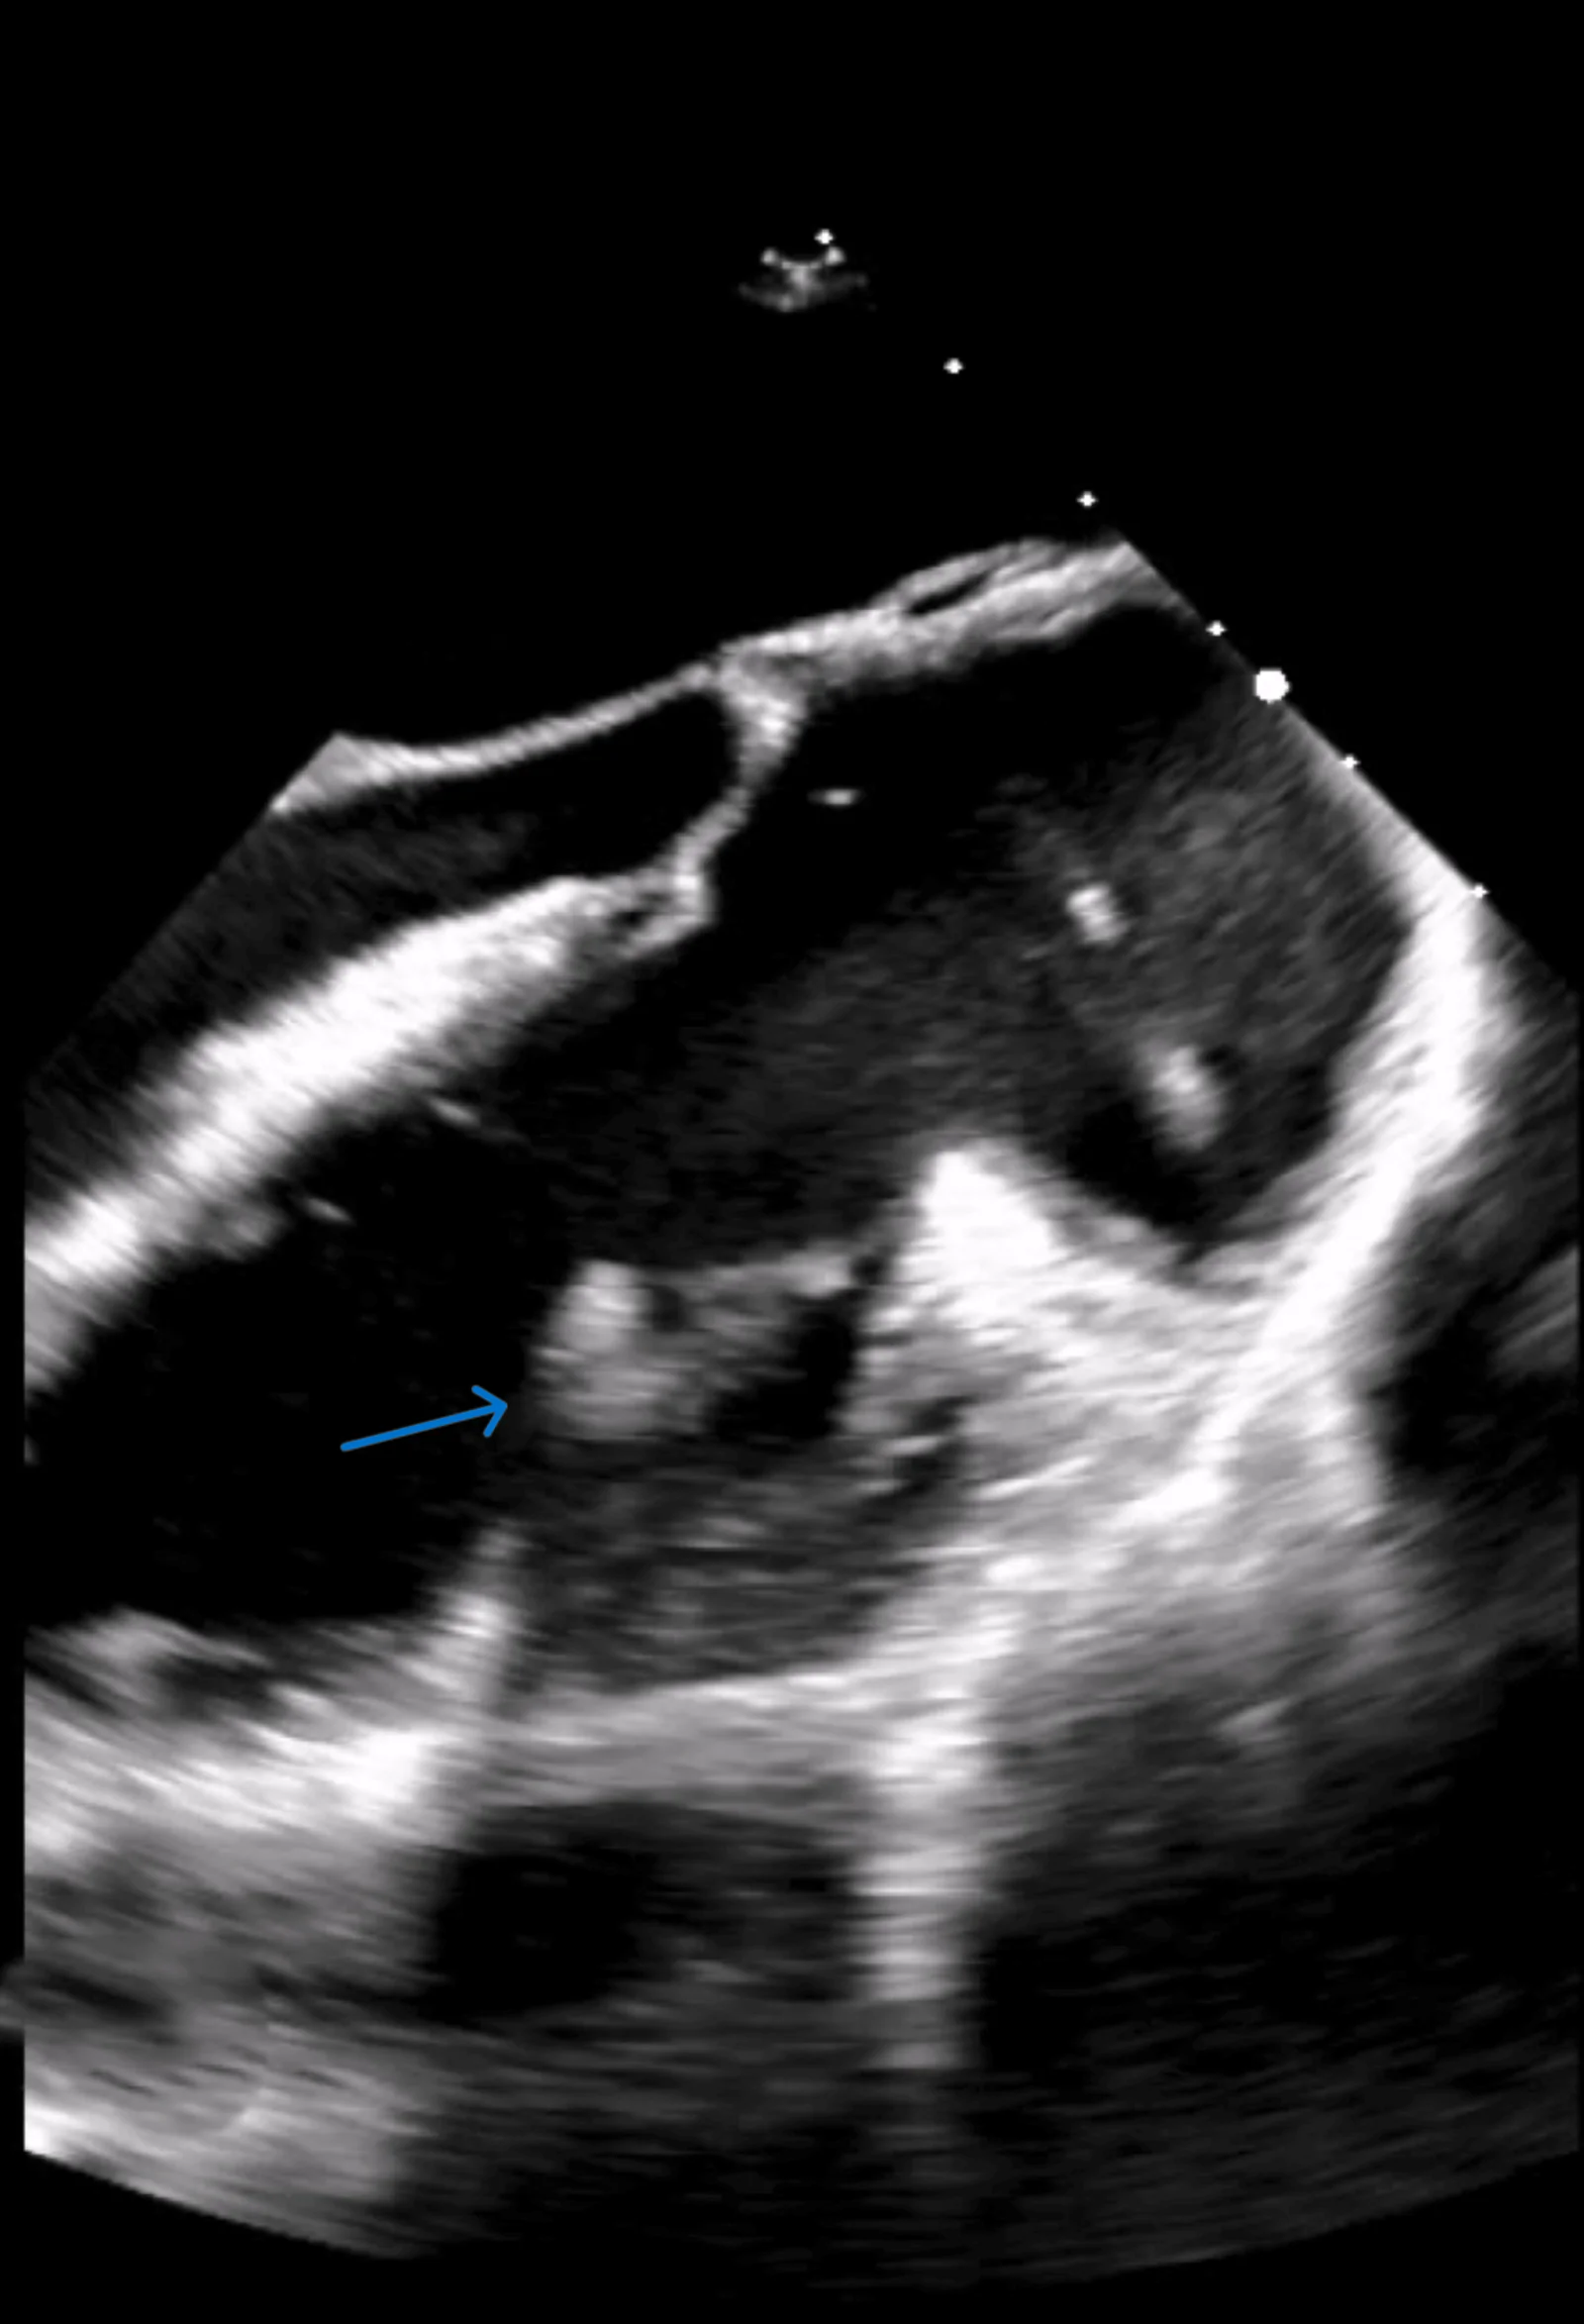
Transthoracic echocardiogram demonstrating a mobile mass consistent with a vegetation on the posterior mitral valve leaflet (blue arrow) (apical 2-chamber view).
